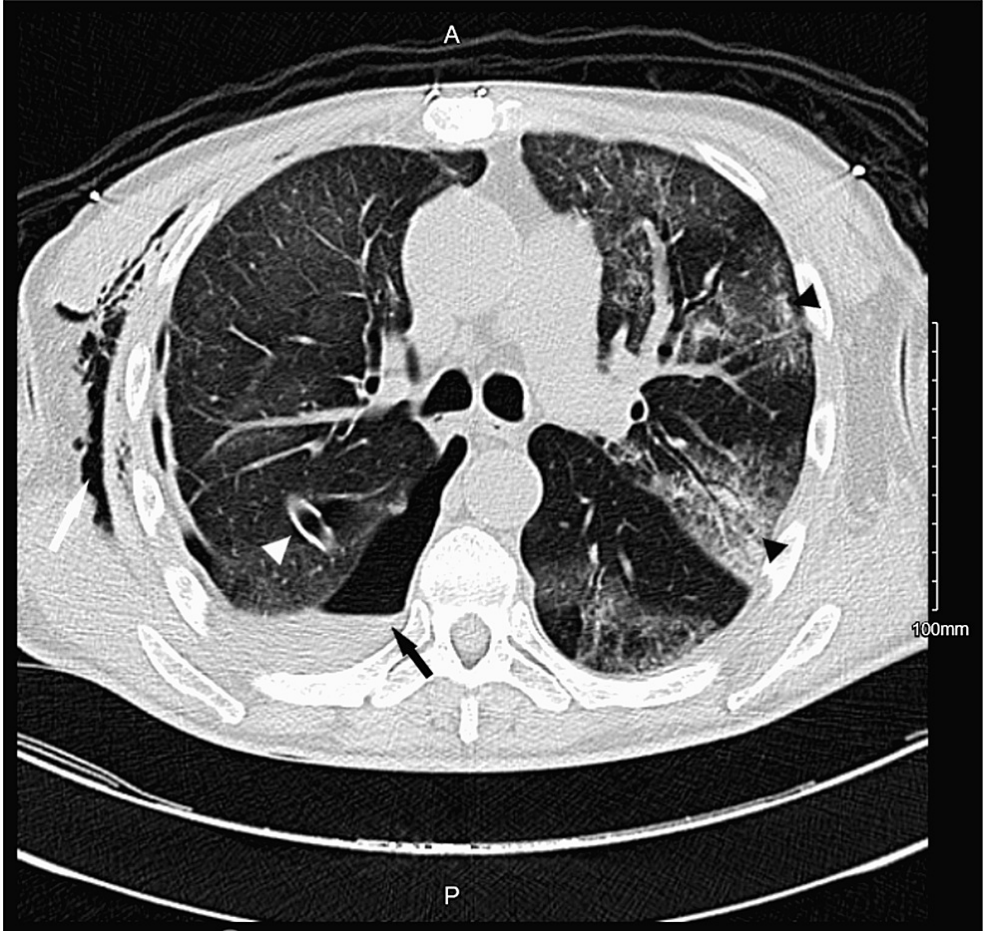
Computed tomography of the chest. Computed tomography of the chest depicting a right hydropneumothorax (black arrow) with associated right lower lobe atelectasis, diffuse bilateral ground-glass opacities suggestive of a pneumonic process (black arrowheads), right subcutaneous emphysema (white arrow), and right-sided chest tube coursing along the right major fissure (white arrowhead).
